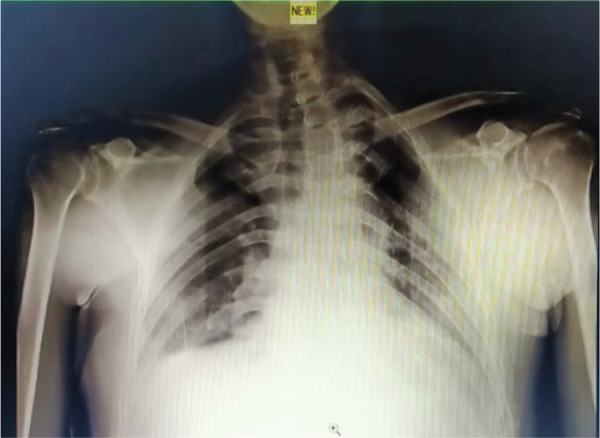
Post close reduction X-ray.
